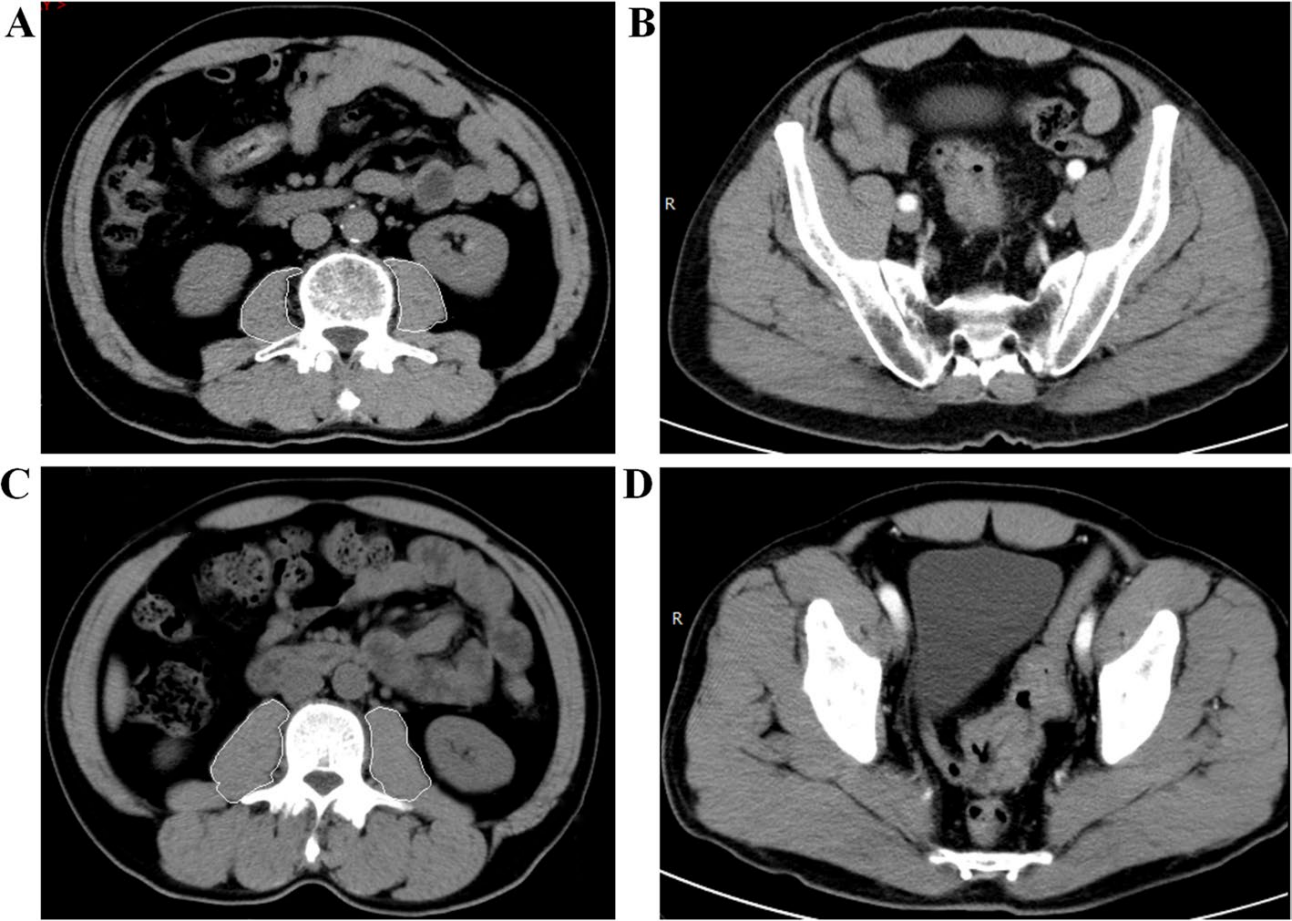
A Measuring the cross-sectional area of the psoas muscles at the level of the third lumbar vertebra for a patient with sarcopenia and sigmoid colon cancer. B Representative CT image of a patient diagnosed with sigmoid colon cancer and sarcopenia. C Measuring the cross-sectional area of the psoas muscles at the level of the third lumbar vertebra for a patient with sigmoid colon cancer but without sarcopenia. D Representative CT image of a patient diagnosed with sigmoid colon cancer but without sarcopenia
